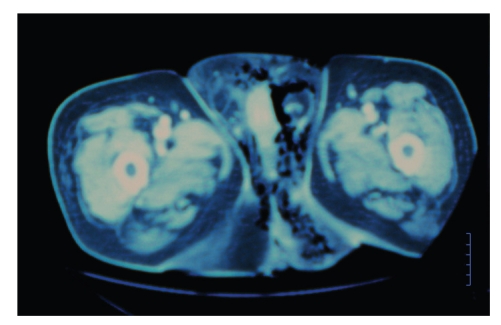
Computed tomography of the pelvis dipecting extensive emphysema around the testicles and perineal subcutaneous tissue.
